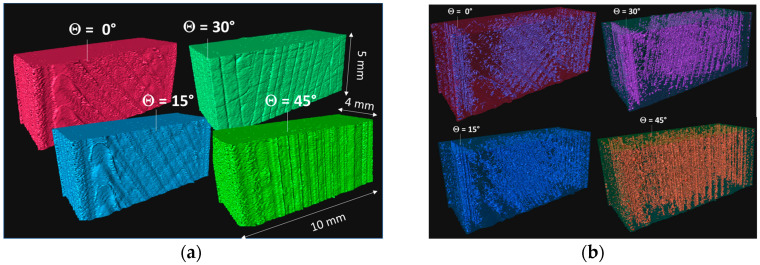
X-ray microtomography results showing (a) a 3D rendering of the overall microstructure and (b) the porous structure as a function of the printing angle (Θ).
